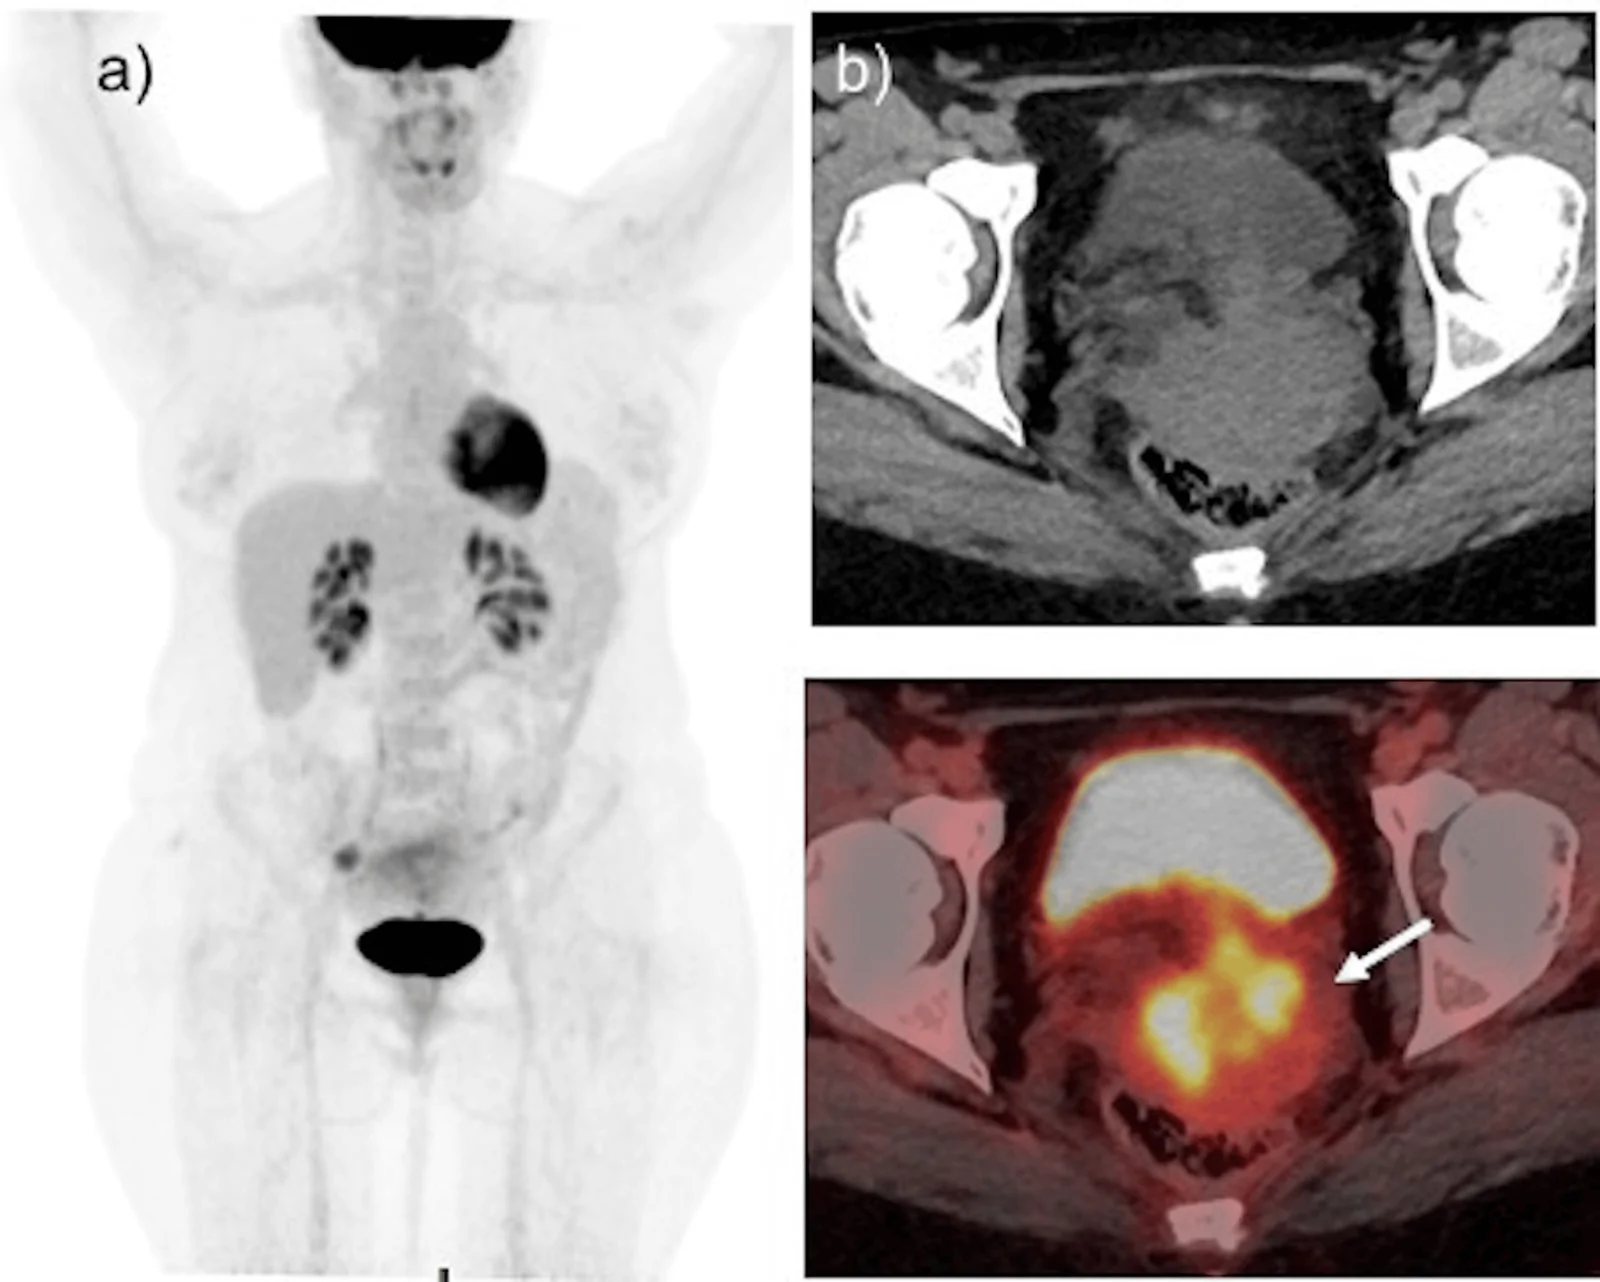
¹⁸F-FDG PET/CT in cervical cancer (a) MIP and (b) axial CT and fused ¹⁸F-FDG PET/CT images demonstrate an intensely ¹⁸F-FDG-avid primary cervical mass (white arrow). No metabolically active regional lymphadenopathy or distant metastatic disease is identified. ¹⁸F-FDG: ¹⁸F-fluorodeoxyglucose; PET: positron emission tomography; CT: computed tomography; MIP: maximum intensity projection
